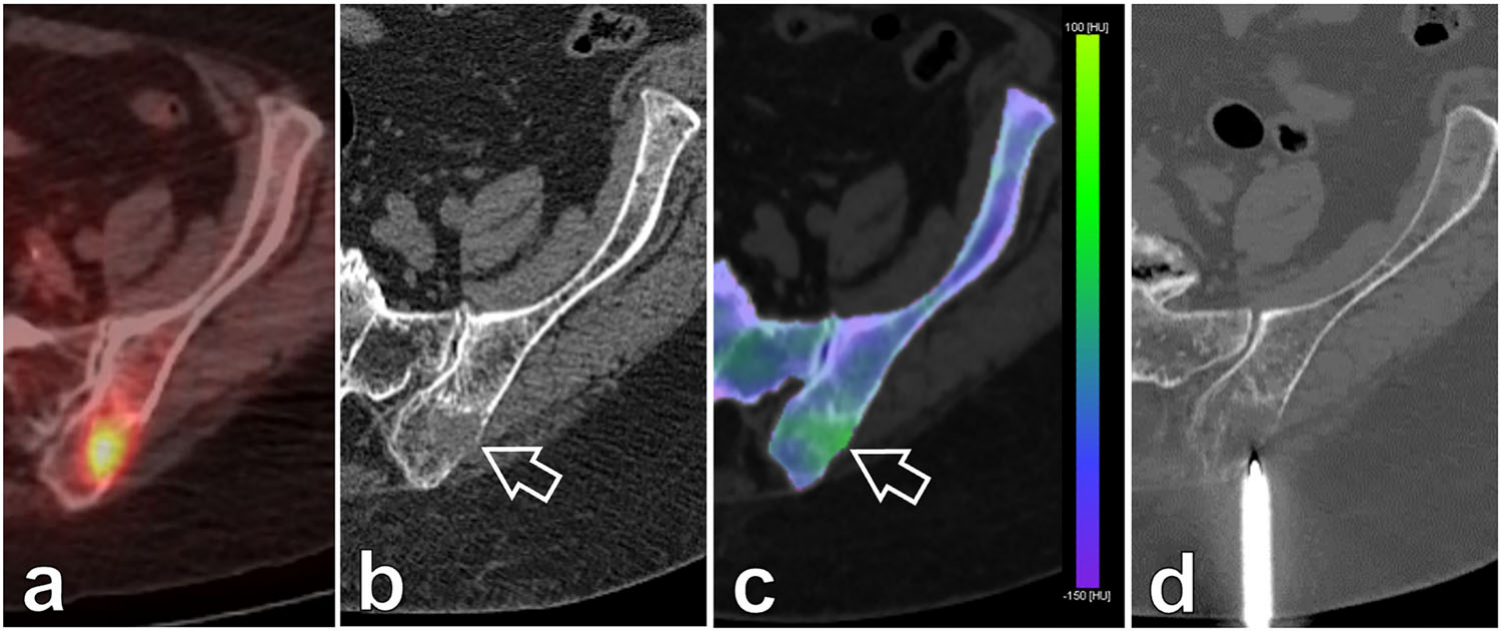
An 89-year-old female patient with a history of stage IV breast cancer was referred for biopsy of a suspicious lesion after a follow-up positron emission tomography/CT (a). b Monoenergetic axial 70-keV bone algorithm reconstruction (0.6 mm thickness; Br80) PCCT-guided biopsy image confirmed the presence of a suspicious lesion (arrow) with a heterogeneous, ill-defined lytic matrix located within the posterior aspect of the left iliac blade. c Real-time BME mapping (2-mm thickness; Qr40) depicted increased and pathological values within the lesion, colour-coded in shades of yellow/green (arrow), while healthy marrow values are colour-coded in blue/violet as seen in the remote iliac bone. d Allowing for an accurate and precise intraprocedural bone biopsy guidance of the target oedematous region with a drilling system. Final diagnosis was breast metastatic disease. BME, Bone marrow oedema; PCCT, Photon-counting computed tomography
